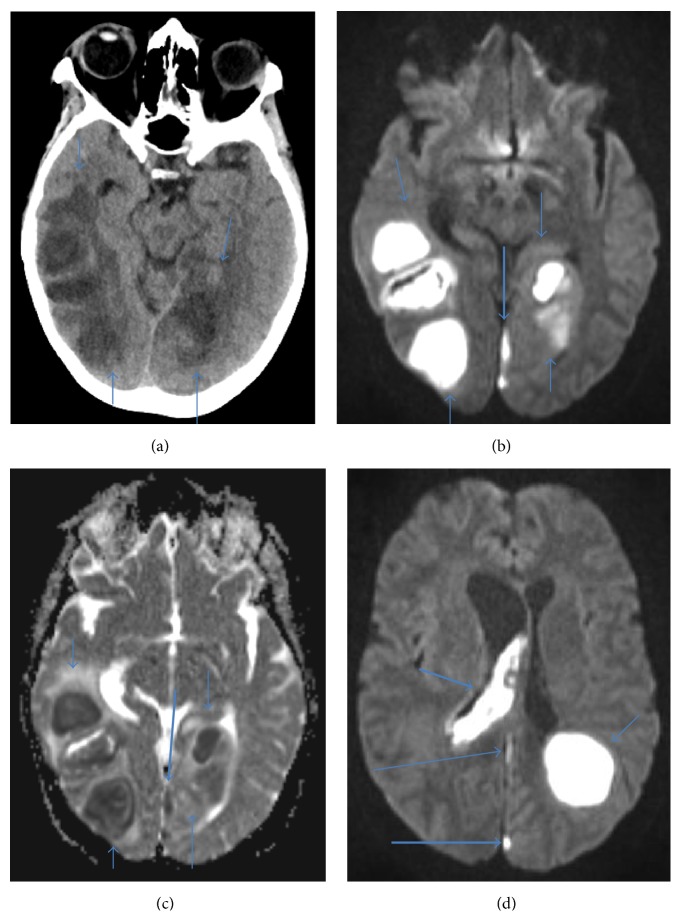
(a) Noncontrast axial CT image, (b) axial diffusion weighted images, (c) axial adjusted diffusion coefficient map that shows bilateral temporal-occipital lobe abscesses (thin arrows) and empyema in the medial left occipital lobe (thick arrow), and (d) axial diffusion weighted image that shows ventriculitis in the right lateral ventricle (short thick arrow), abscess in the left parietal lobe (short thin arrow), and empyema in the posterior falx (long thin arrow) and medial left occipital lobe (long thick arrow).
